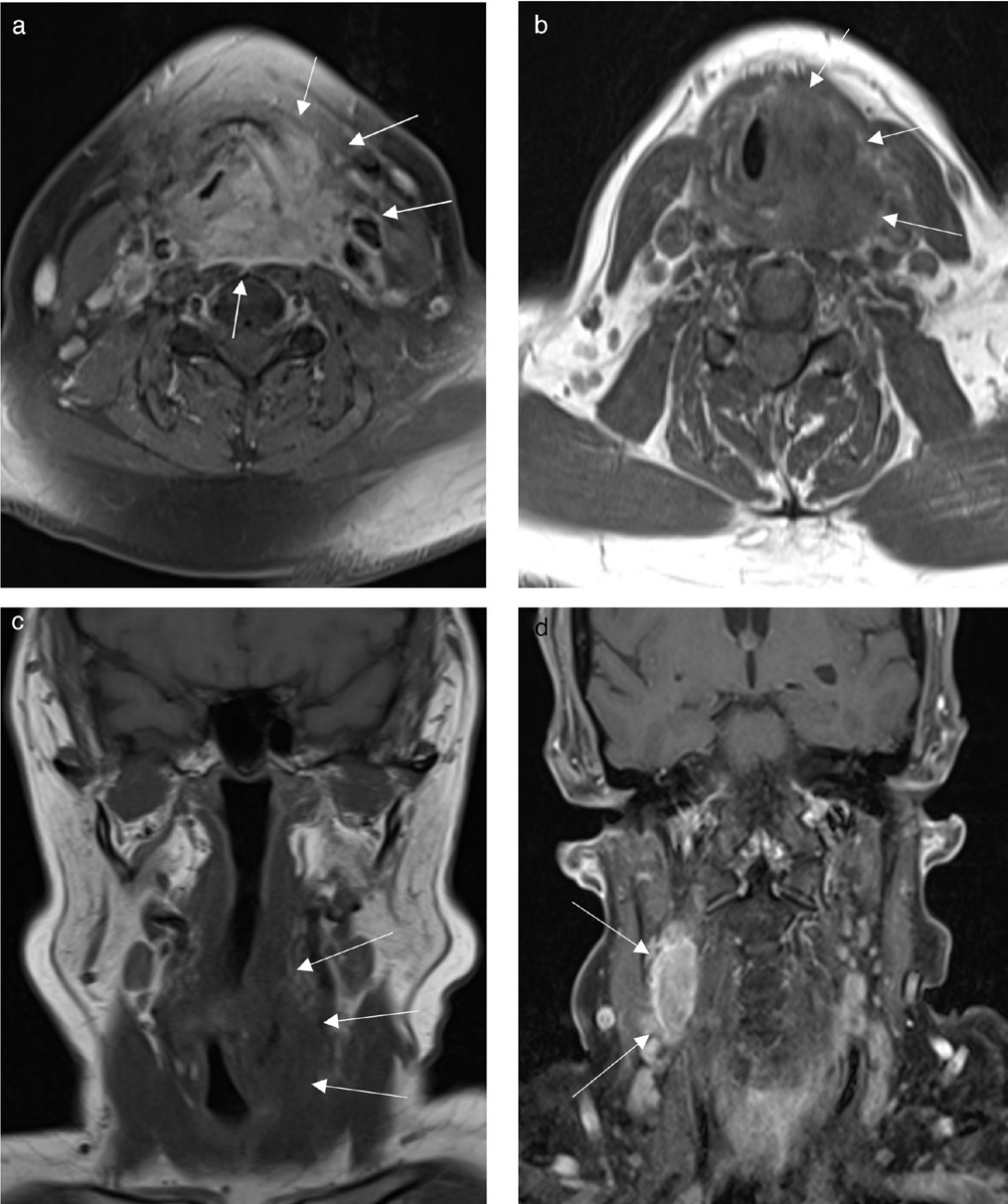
(a) Axial MRI fat saturated T1 post-contrast image shows an enhancing infiltrative mass involving the left glottis which appears thickened and displaced to the right side. This abuts the left carotid sheath, extending posteriorly into the retrovisceral space and crosses the midline to the right side (arrows). (b) Axial MRI T1 image shows infiltration of the left sternohyoid muscle and anterior cervical space. (c) Coronal MRI T1 image shows extension along the posterolateral hypopharyngeal wall, inferiorly inseparable from the left superior thyroid lobe. (d) Coronal MRI fat saturated T1 post-contrast image shows an enlarged enhancing right level two lymph node.
